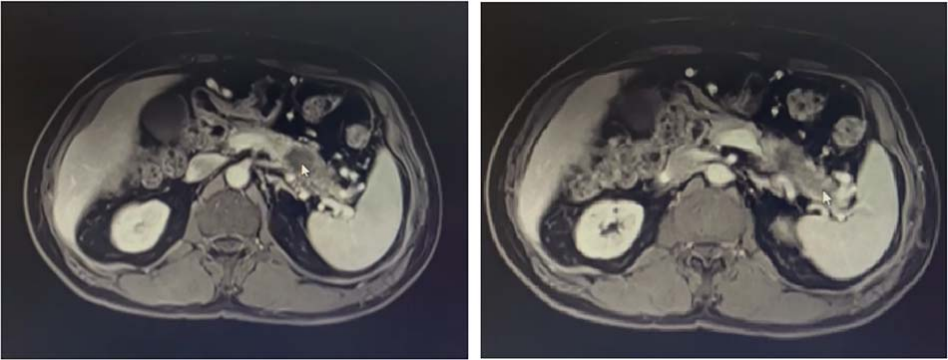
Metastatic masses can be seen in the pancreatic tissue on the CT-Scan (transverse/axial view)
